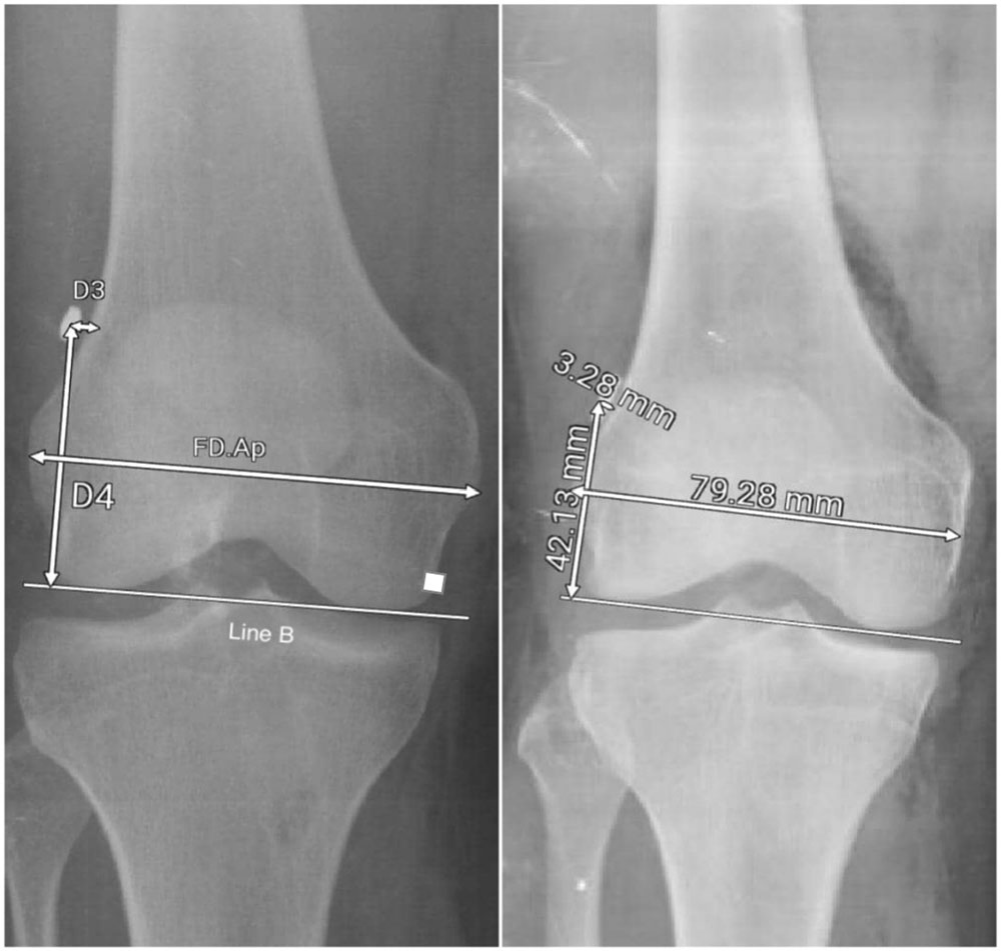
Features of anteroposterior radiographs.
